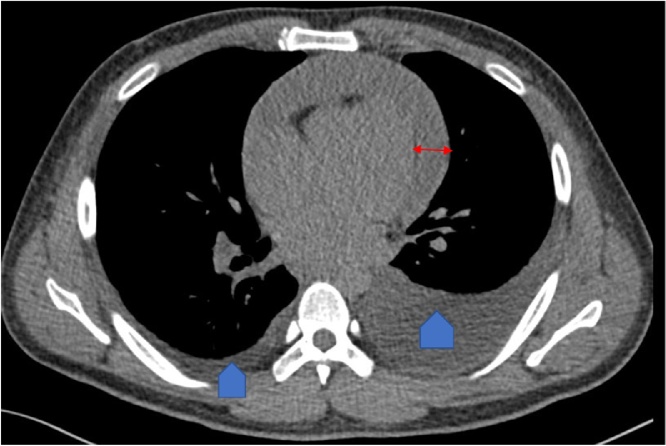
Chest CT upon recent admission. Congestive pulmonary changes in the form of ground glass opacities and pleural effusion at the posterior inferior aspects of both lungs, more on the left. (Arrow heads) Circumferential pericardial effusion (red arrow).
